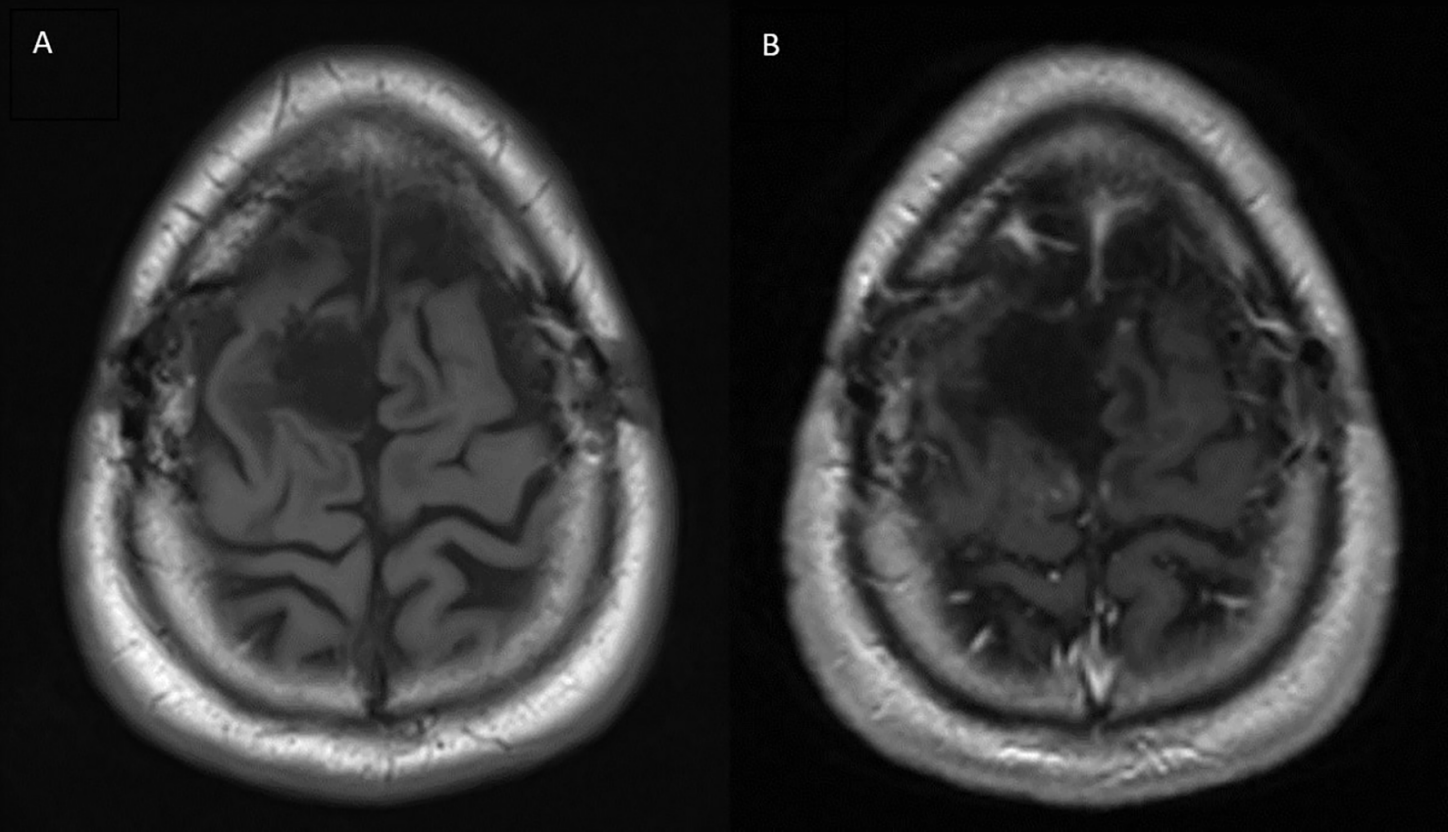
Brain MRI performed 34 months after completion of radiotherapy treatment. (A) T1 pre-contrast (B) T1 post-contrast showing no areas of peripheral or nodular enhancement.
